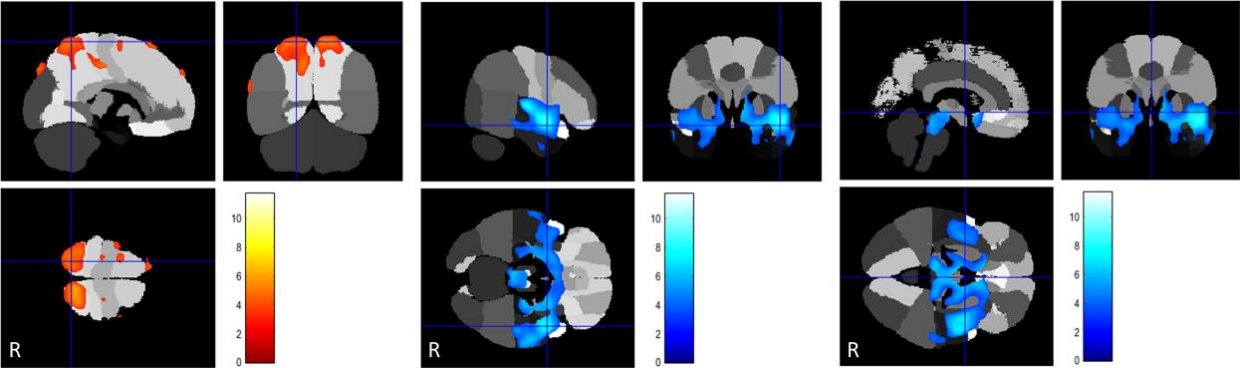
FDG uptake in CP-preterm cases (*n* = 35) compared to controls. *Left*: Relative hypermetabolism in the superior parietal lobes displayed in a yellow-scale with brighter tones indicating higher *t*-scores. *Middle* and *right*: Areas of relative hypometabolism, involving the superior-medial temporal lobes, peri-insular cortex, globi pallidi, thalami and brainstem, displayed in a blue-scale with brighter tones indicating higher *t*-scores.
